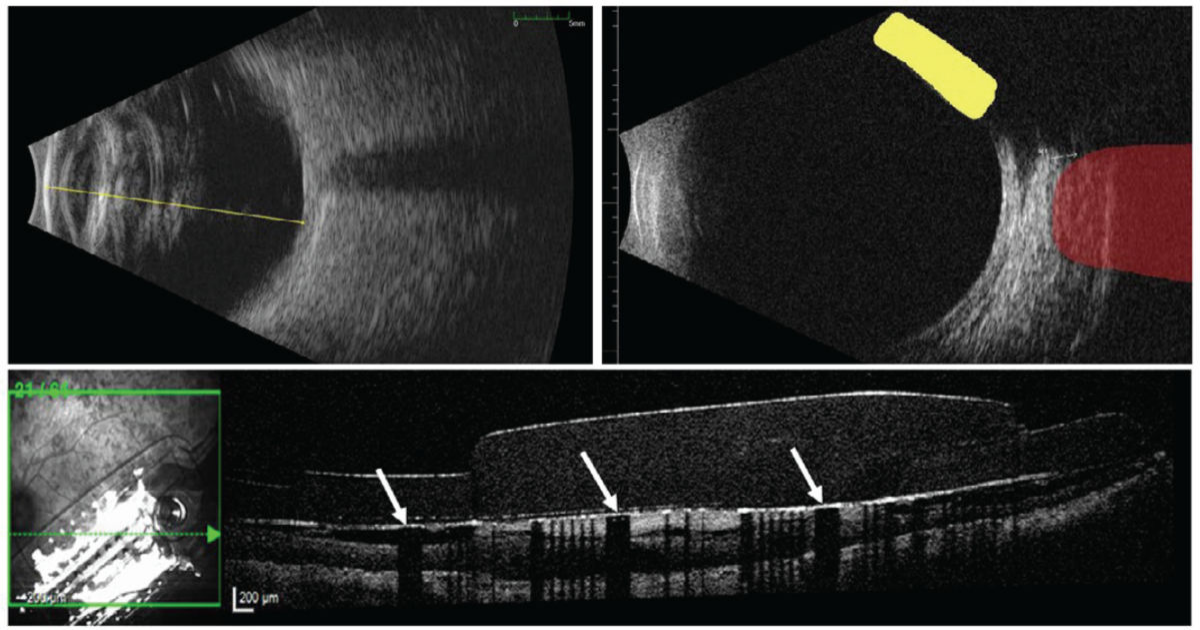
Ultrasound and OCT. The ultrasound on the top left shows axial length
